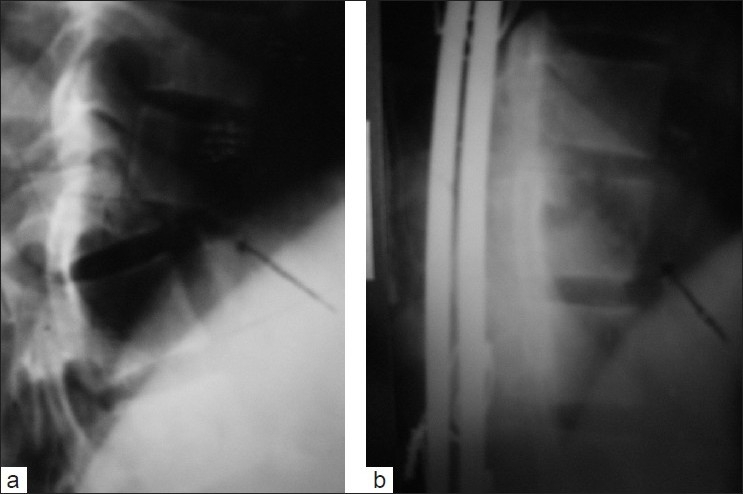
(a) A lateral radiograph of dorsolumbar spine of a 22-year-old male patient shows fracture dislocation of D11 with severe kyphotic deformity along with fracture of superior end plate of D11 vertebral body (arrowhead), presented with complete paraplegia. (b) Harrington rod and sublaminar wiring fixation done extending 2-level cephalad and 3-level caudalad demonstrating excellent correction of kyphosis and restoration of the vertebral height
